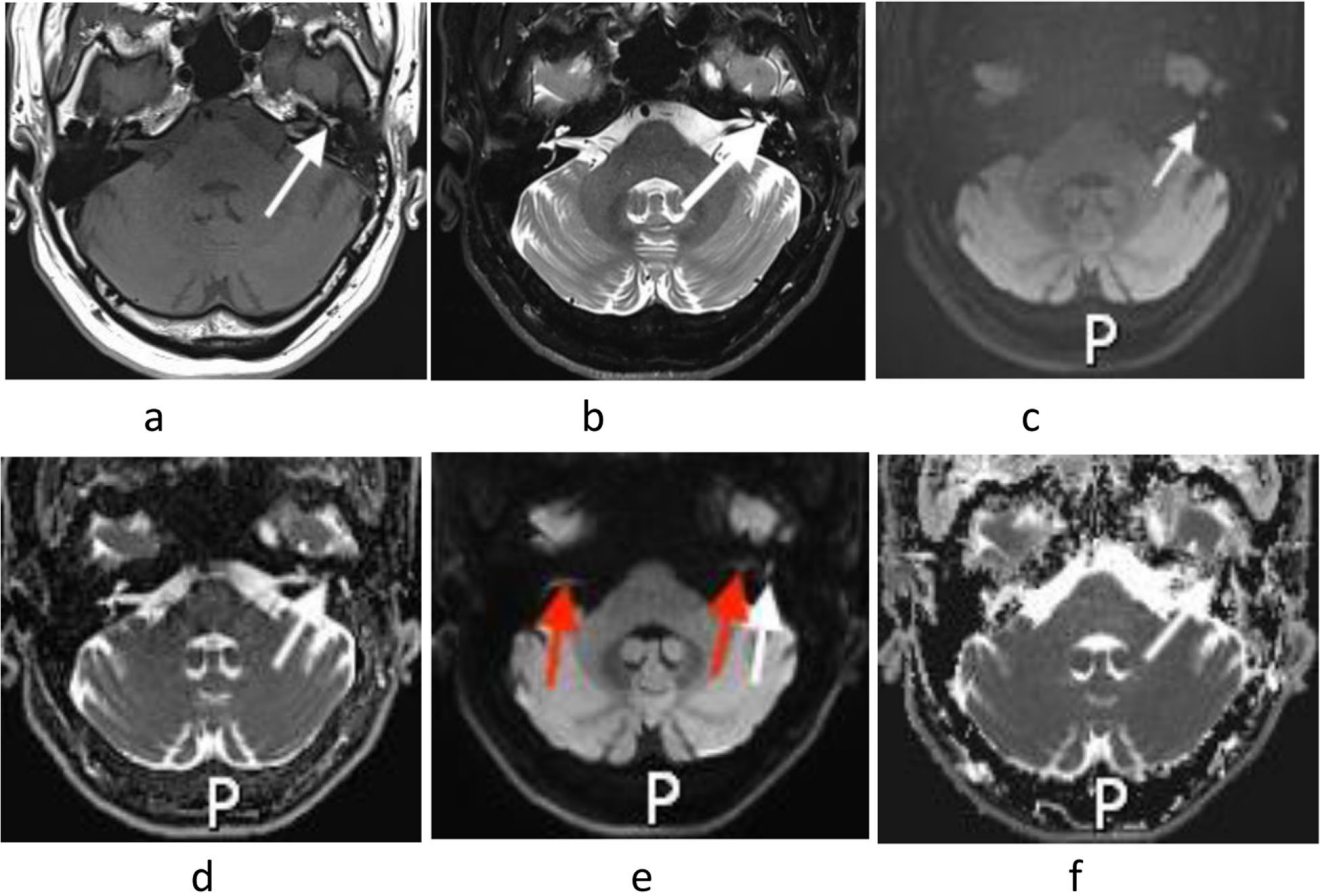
A 44-year-old male with a small cholesteatoma (1.9 mm width) in the left tympanic cavity (white arrow). a: Axial T1WI. b: Axial T2WI showing the structure of the small cholesteatoma (white arrow). c: Axial TGSE BLADE DWI (b1000) clearly showing a markedly high signal intensity for the small cholesteatoma (white arrows) without artifacts. e: Axial RESOLVE DWI (b1000) showing the high signal intensity of a small lesion (white arrow) with light geometric distortion and the bilateral middle ear mastoid process with a few artifacts (red arrow). d, f: The ADC values on TGSE BLADE and RESOLVE DWI were 0.737 × 10^− 3^ mm^2^/s and 0.984 × 10^− 3^ mm^2^/s, respectively
